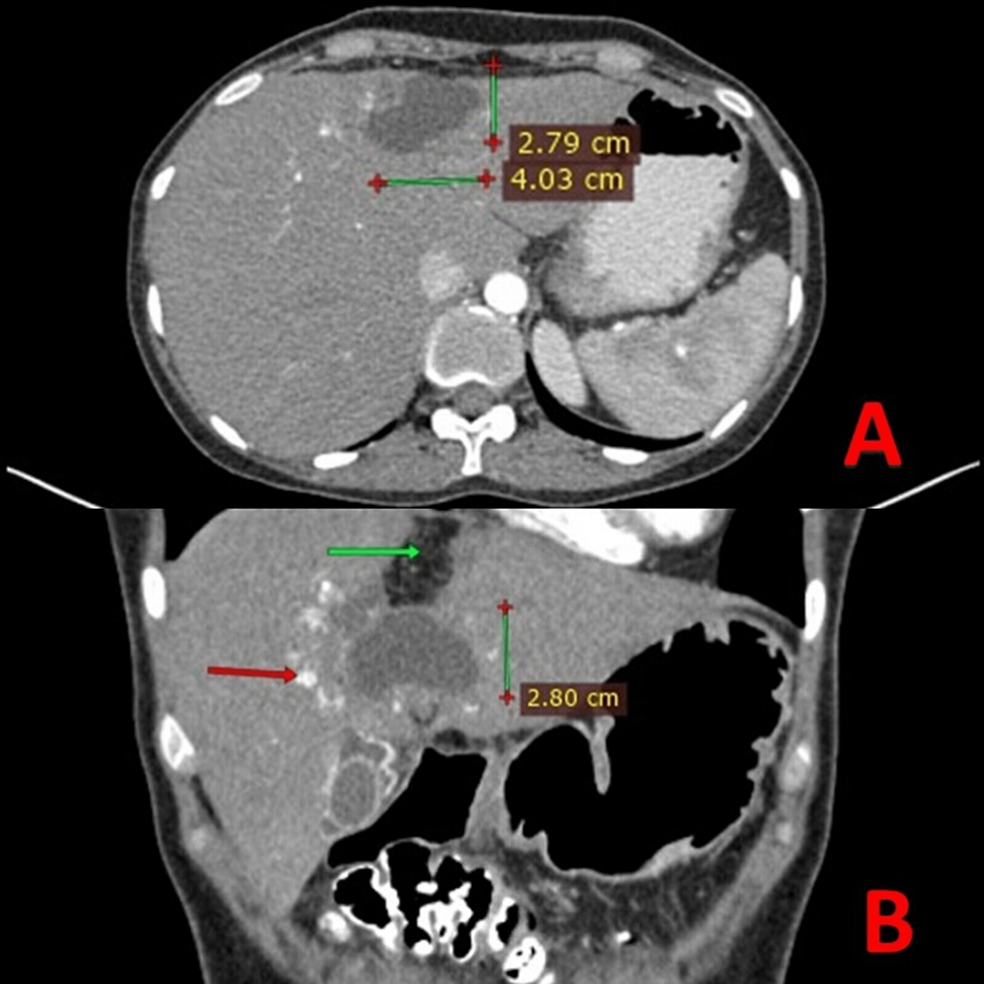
Postoperative contrast-enhanced CT scan (CECT) (A) Axial plane-Arterial phase showing residual cystic lesion in segment IV-a of the liver. (B) Coronal plane showing peripheral foci of calcifications (red arrow) and postoperative omental packing (green arrow).
